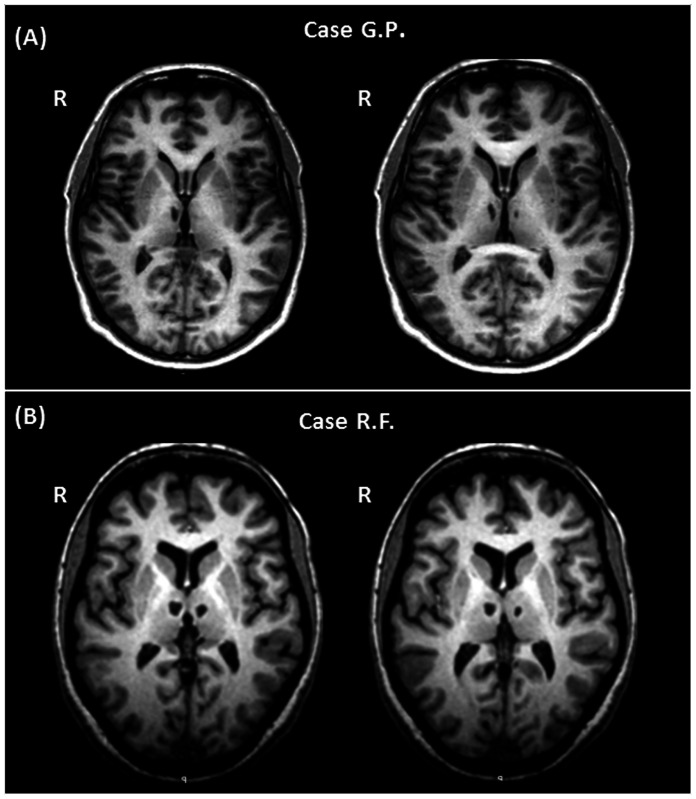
Macroscopic thalamic damage in the two studied patients. Bilateral thalamic damage detectable on T1-weighted images of the two patients. G.P. shows a more asymmetrical involvement of the thalamus, with the right lesion considerably larger than the left one (A). Conversely, R.F. presents with a more symmetrical thalamic involvement, with both lesions showing a similar location and extension (B).
